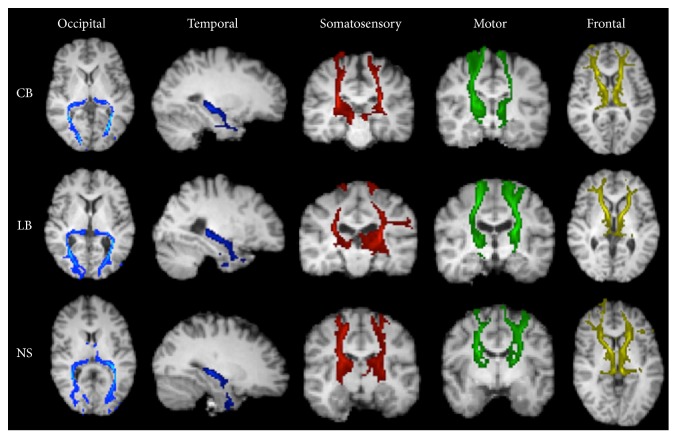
Examples of cortical white matter projections from each of the five thalamic clusters to the cortical target masks in a CB, LB, and NS participant. The cortical target masks are defined from the Desikan-Killiany atlas provided with FreeSurfer, as described in Table 1. The tracts are overlaid on each subject's T1-weighted image and presented in a slice of the most representative view, axial or coronal.
